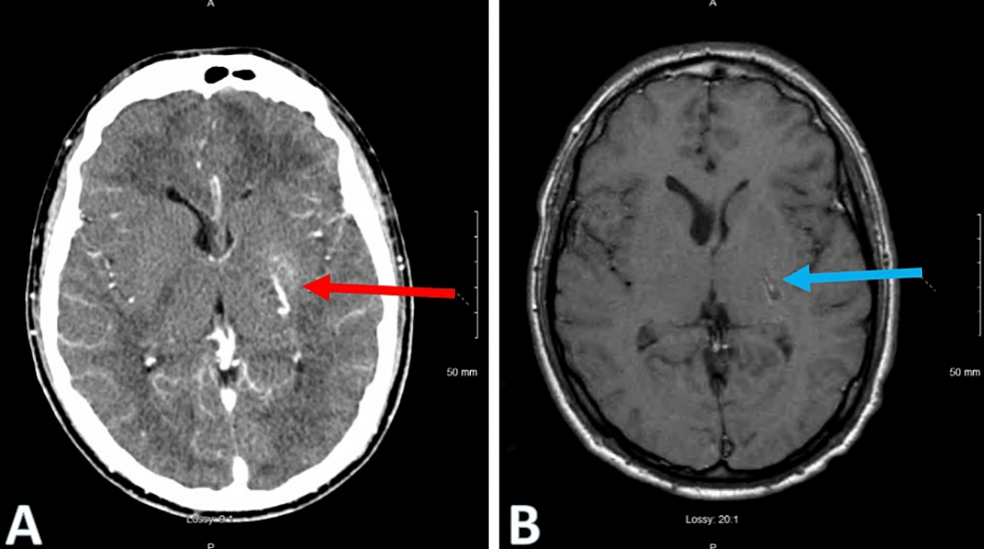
Computed tomography (CT) and magnetic resonance imaging (MRI) of the brain A. CT scan of the head with IV contrast suggestive of venous vascular malformation in the left basal ganglia (red arrow). B. MRI of the brain without IV contrast consistent with a 2 cm nidus of venous malformation in the left basal ganglia (blue arrow).
